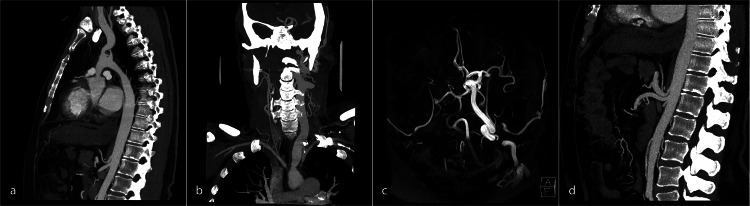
CT thoracic angiogram, head, carotid and circle of Willis angiogram.
